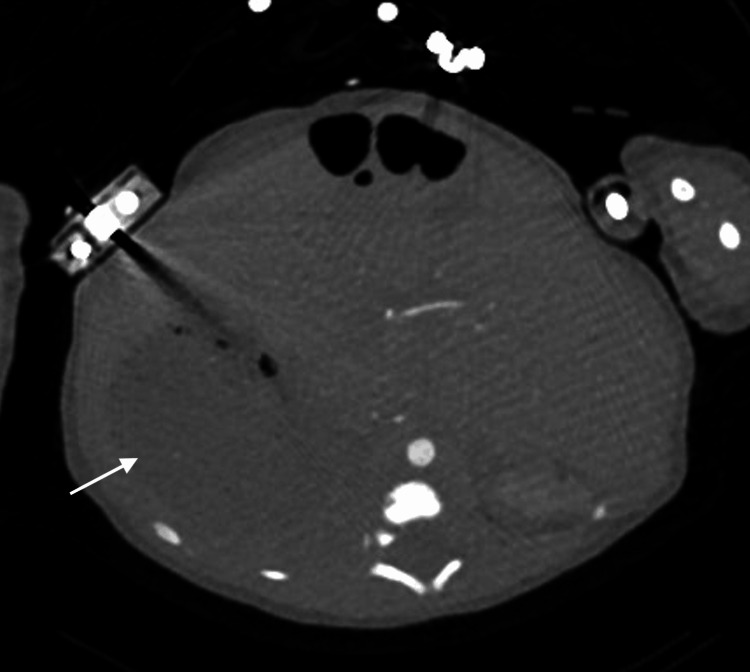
Axial contrast-enhanced computed tomography image demonstrating a right-sided stomach (white arrow)
